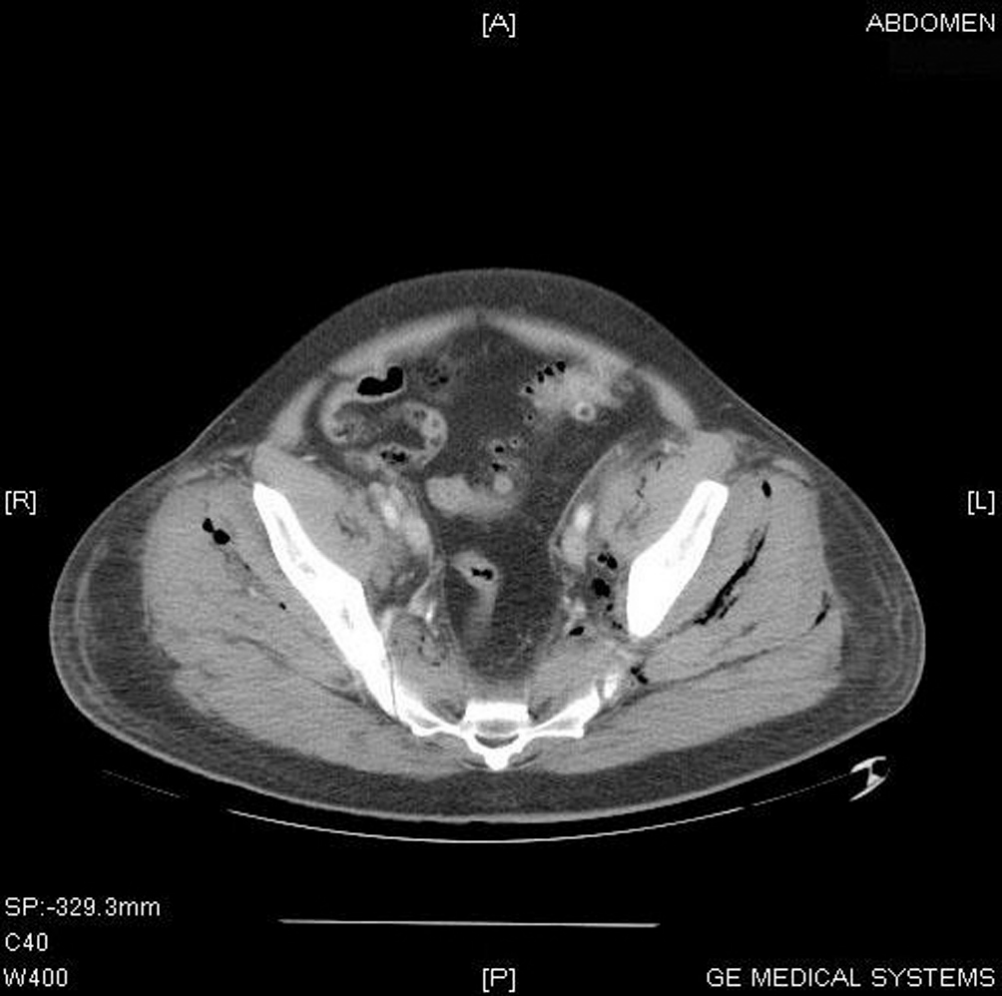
CT of the pelvis demonstrating extensive air and fluid extending through the pelvic fascias to the pelvic muscles.
